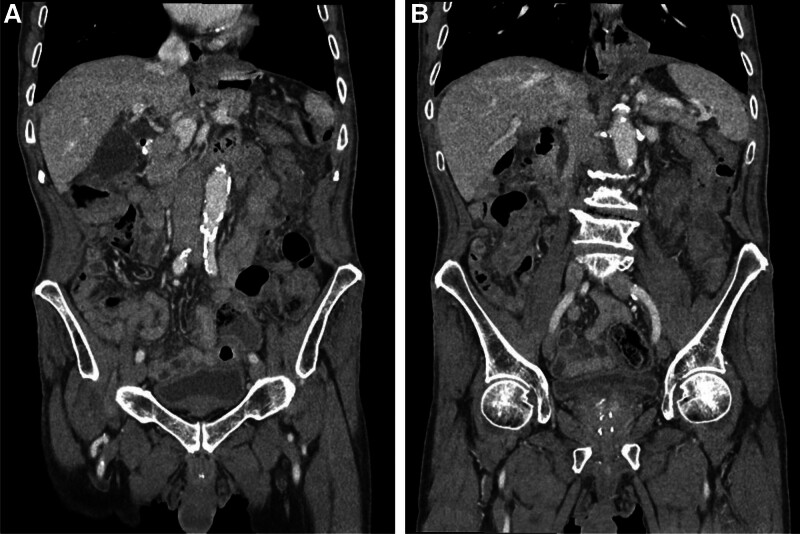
Abdomen–pelvis computed tomography after surgery revealing: (A) Resolved hiatal hernia and decompression of the afferent loop. (B) Mild dilation of extrahepatic bile duct.
